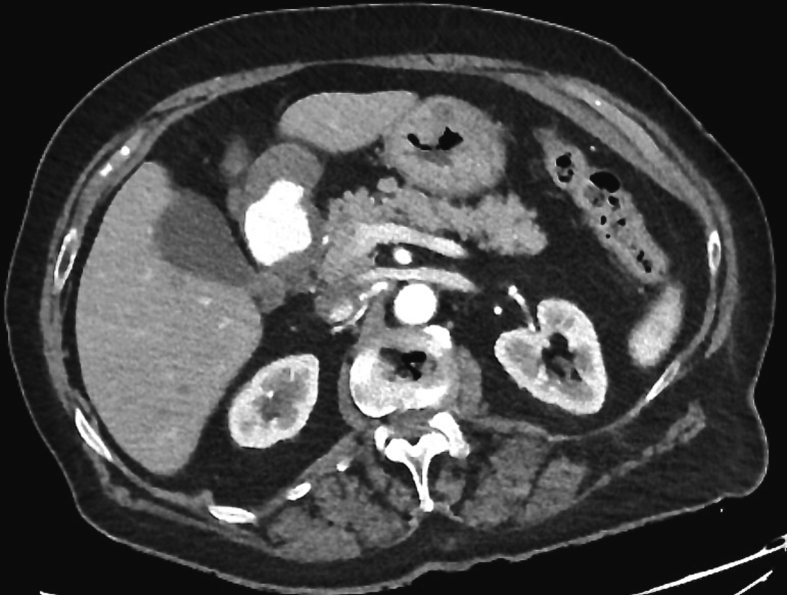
CT scan of the patient's abdomen demonstrating evident contrasts extravasation.
